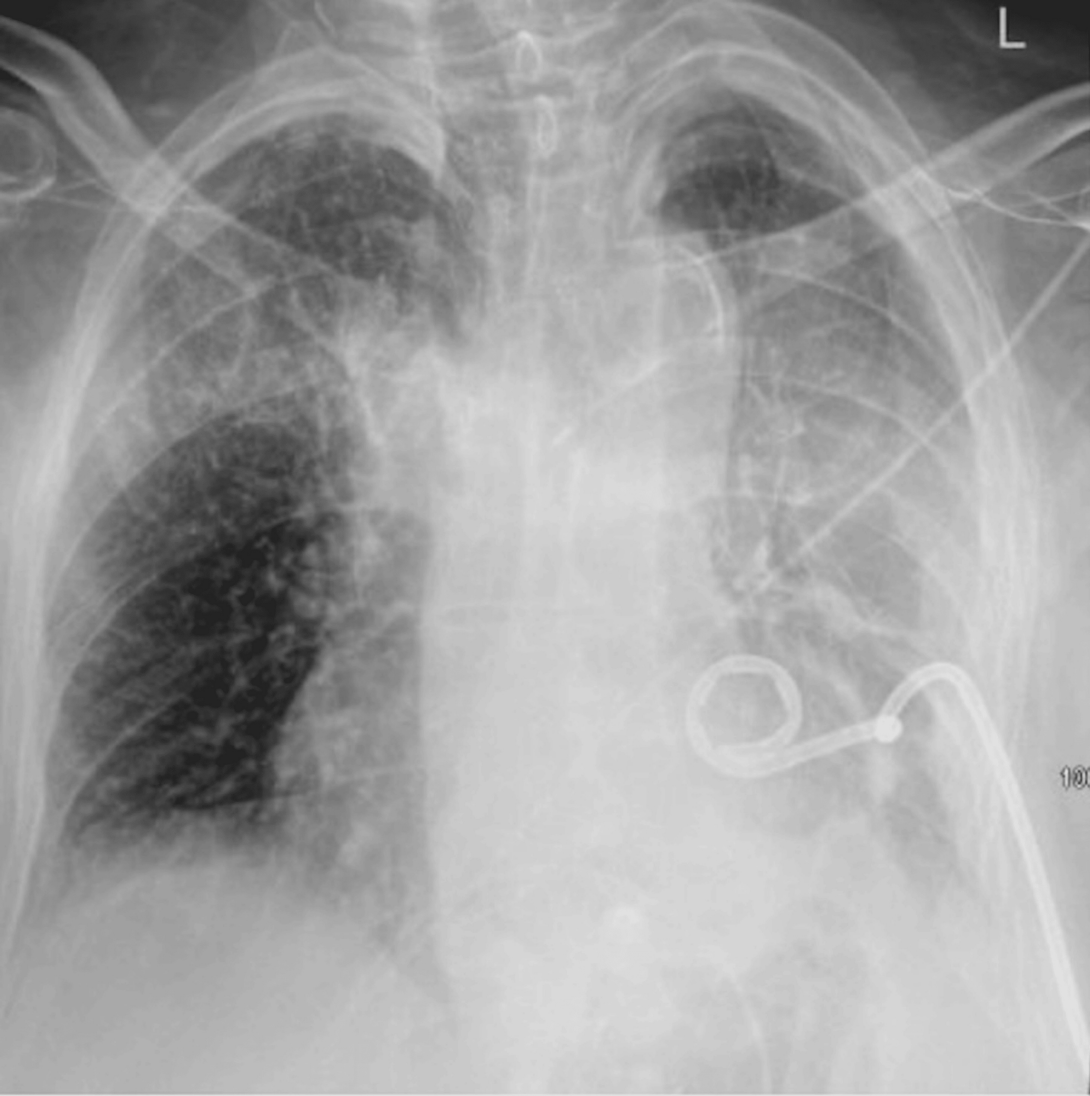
An anteroposterior (AP) chest x-ray demonstrates near-complete resolution of the left hemothorax after intrapleural administration of tPA and DNase. The left lung shows significant re-expansion and improved aeration, now appearing symmetrical to the right lung.
